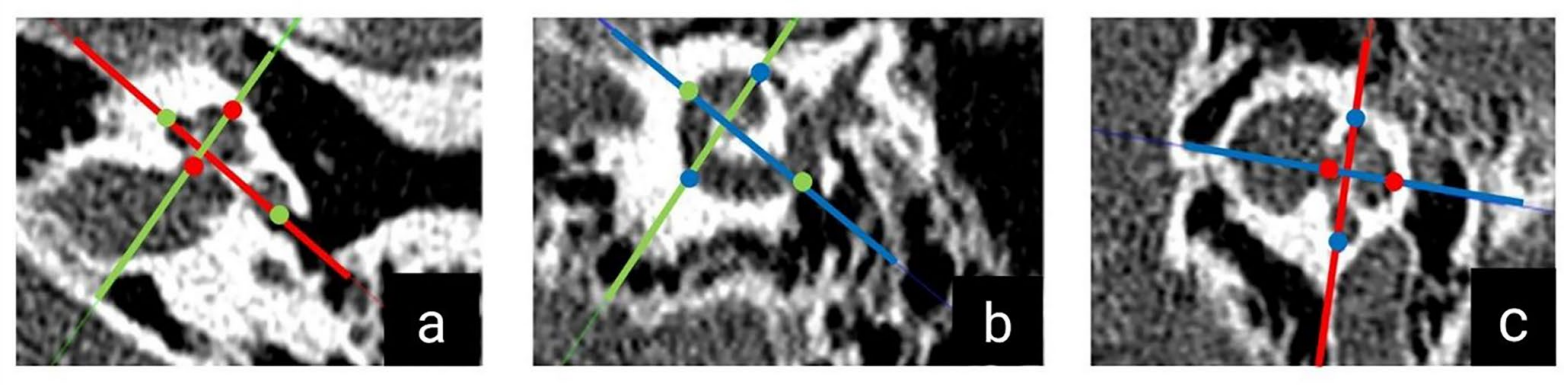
CT images in the OTOPLAN visualisation. The coloured markings were placed by the evaluator and show the diameter (green dot on red line / A value), the width (blue dot on red line / B value) and the height (red dot on green line / H value) of the cochlea. Axial, coronal and sagittal CT views are shown respectively in a, b, and c
